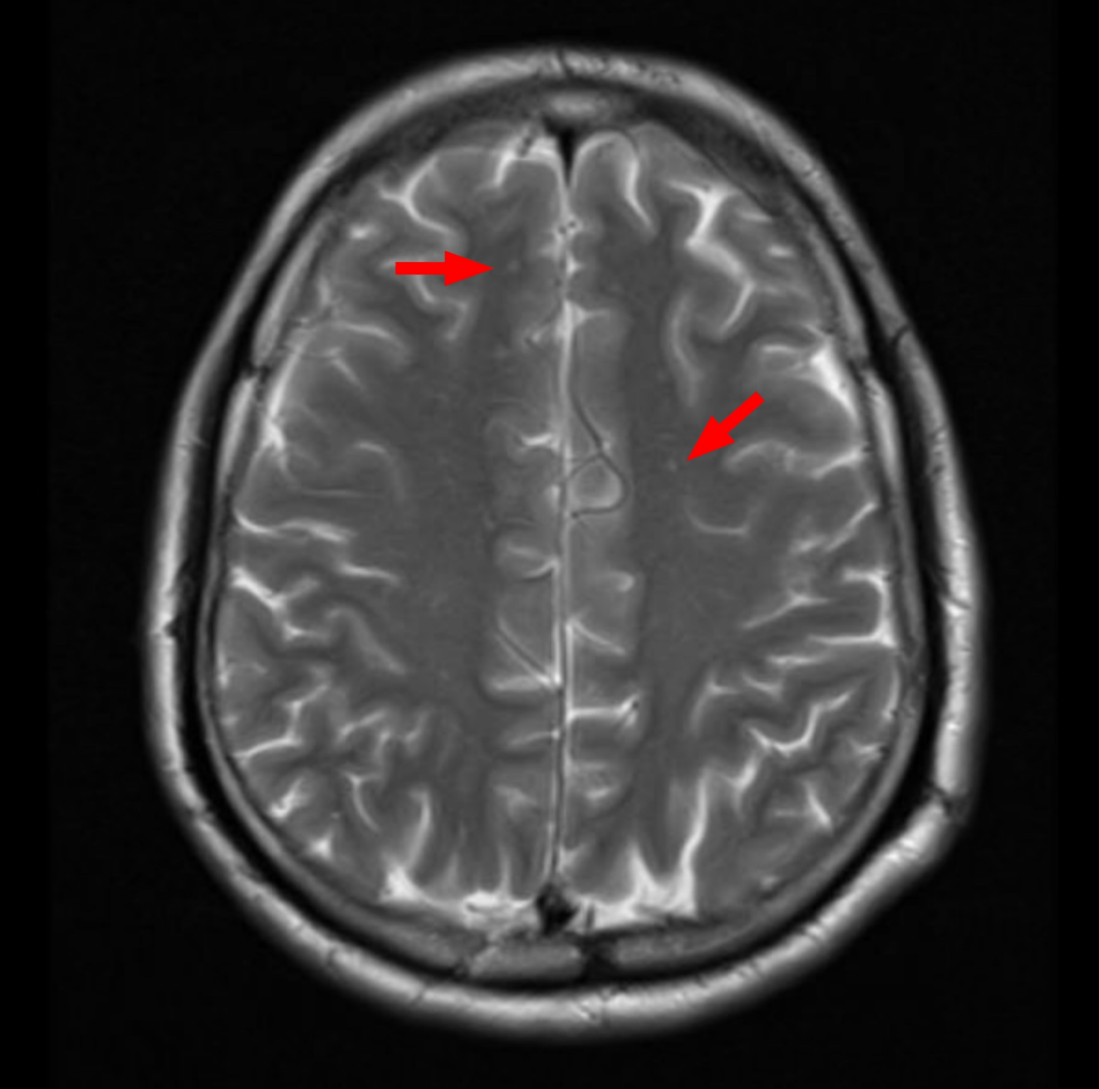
Magnetic resonance imaging (MRI) of the brain Red arrows: multiple small, high signal foci in white matter.
